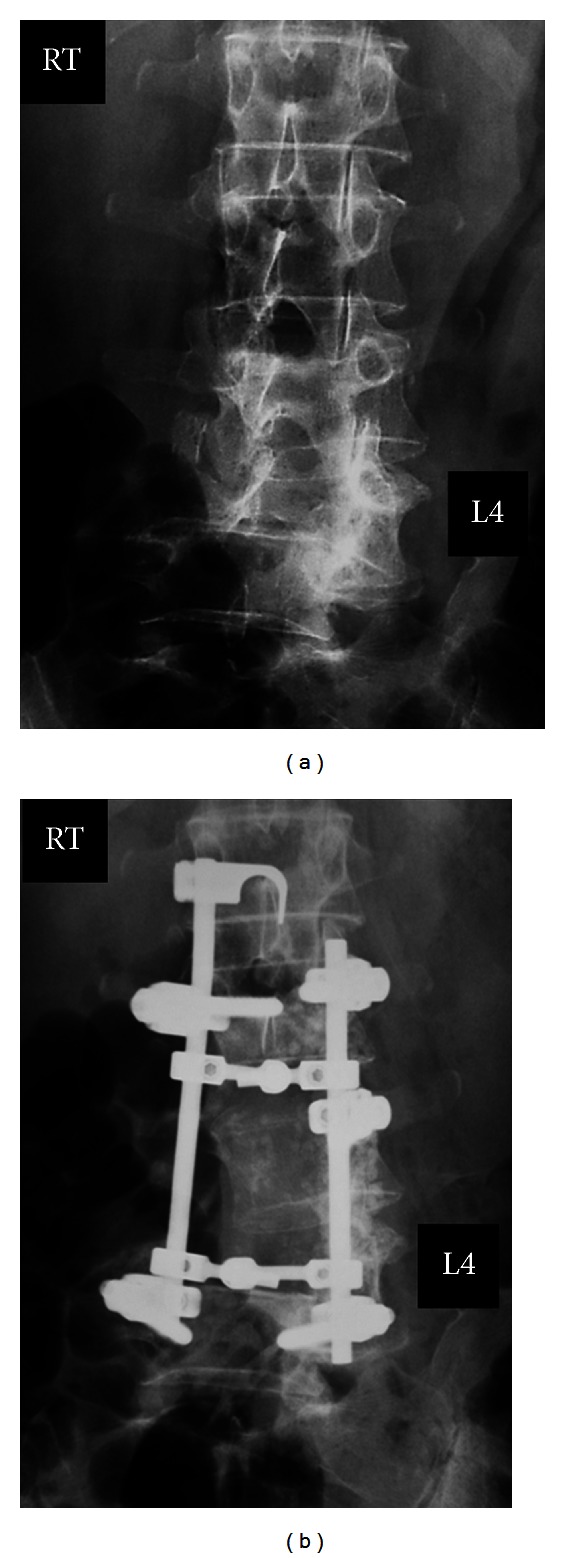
Plain radiographs before and after the first surgery (January 2007). Six months after the first consultation, the tumor size was increased and had invaded the entire L4 and right L3 vertebral body (a). Spine reconstructive surgery was conducted using a pedicle screw, hook-and-rod system (b).
